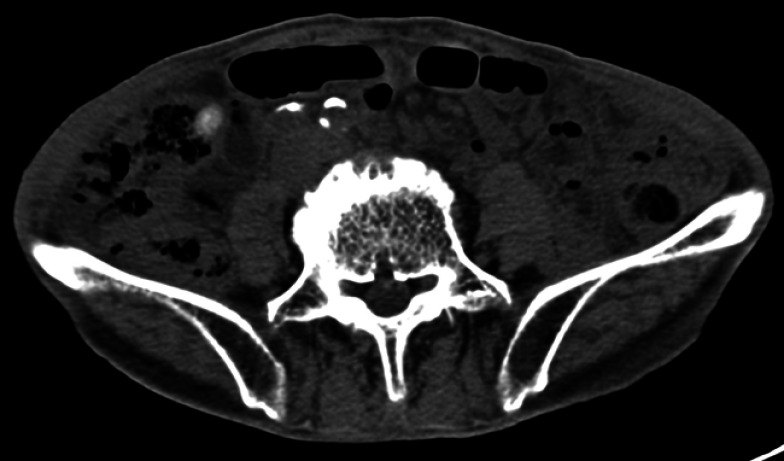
Abdominal computed tomography scan demonstrating a localized high-density area in the appendix.
